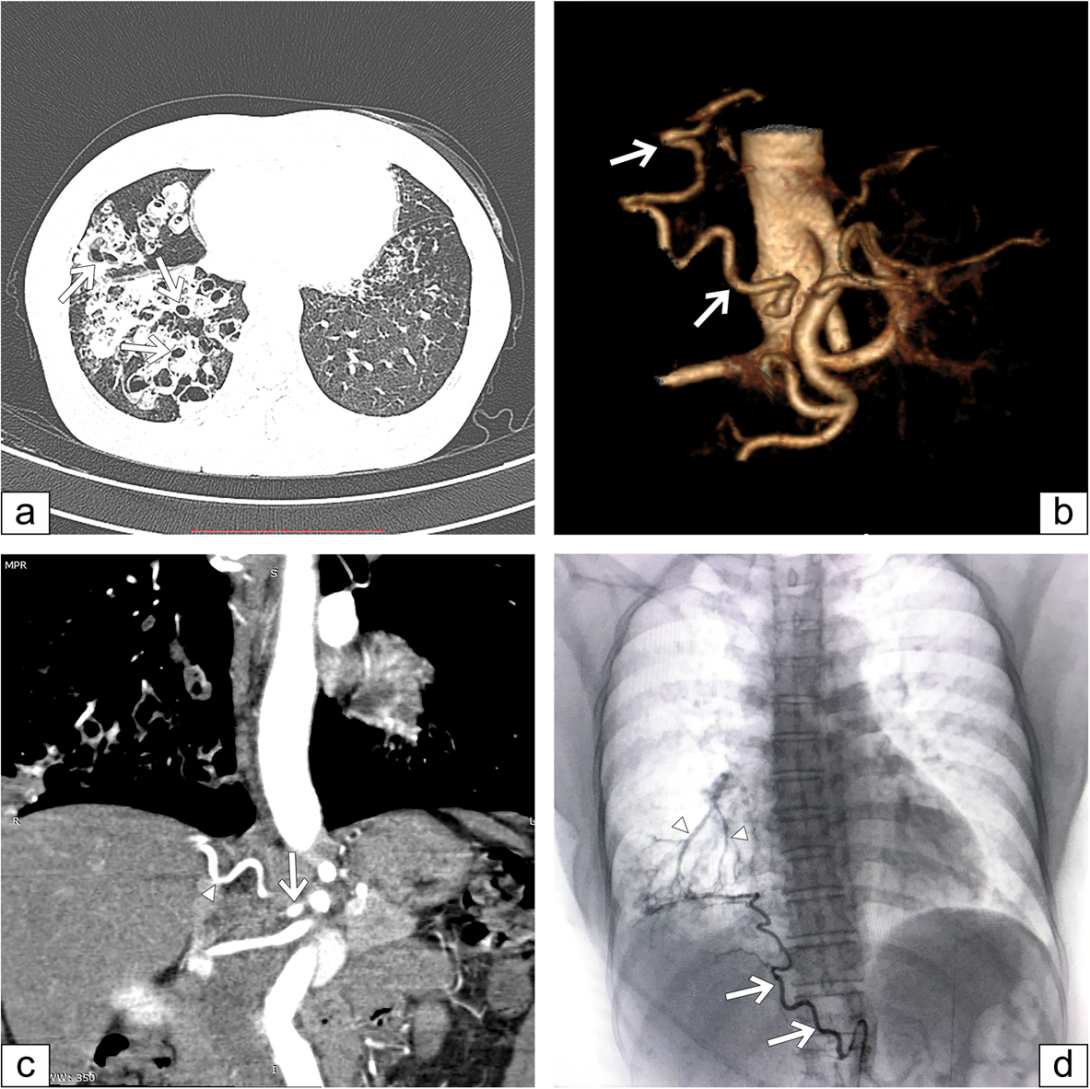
A case presentation with moderate hemoptysis. a A chest computed tomography scan demonstrates multifocal bronchiectasis that involves the middle and lower lobes of the right lung *(arrows).*
